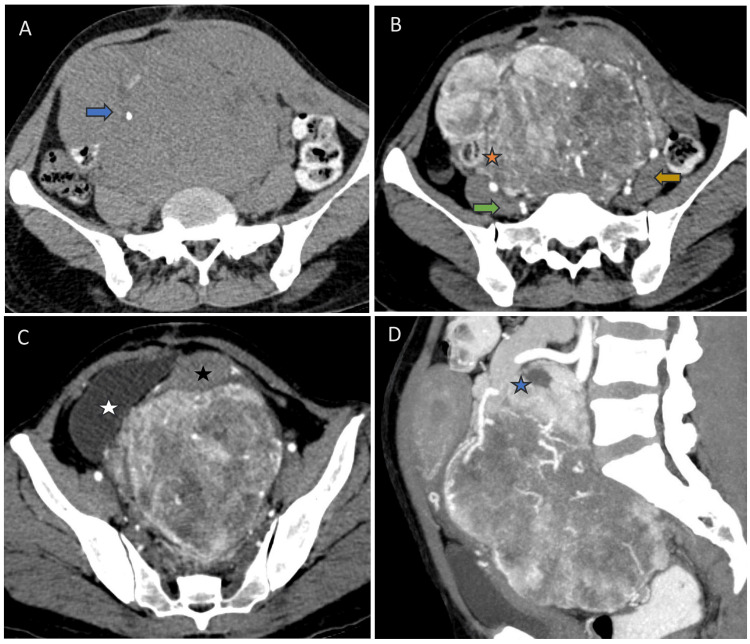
CT images. (A) Unenhanced axial scan reveals a multilobulated abdominopelvic solid lesion centred in the sigmoid mesentery with few coarse calcifications (blue arrow). (B) Contrast-enhanced axial scan in the arterial phase shows the lesion abutting the iliac arteries posteriorly with maintained fat planes (green and orange arrows). The sigmoid colon is compressed and displaced by the lesion towards the right side with no signs of obstruction (orange star). (C) The lower axial sections in arterial phase reveal the lesion abutting and displacing the uterus anteriorly (black star) and the urinary bladder to the right side (white star) with maintained fat planes. (D) Sagittal view in arterial phase shows the lesion supplied by the inferior mesenteric artery (blue star).
